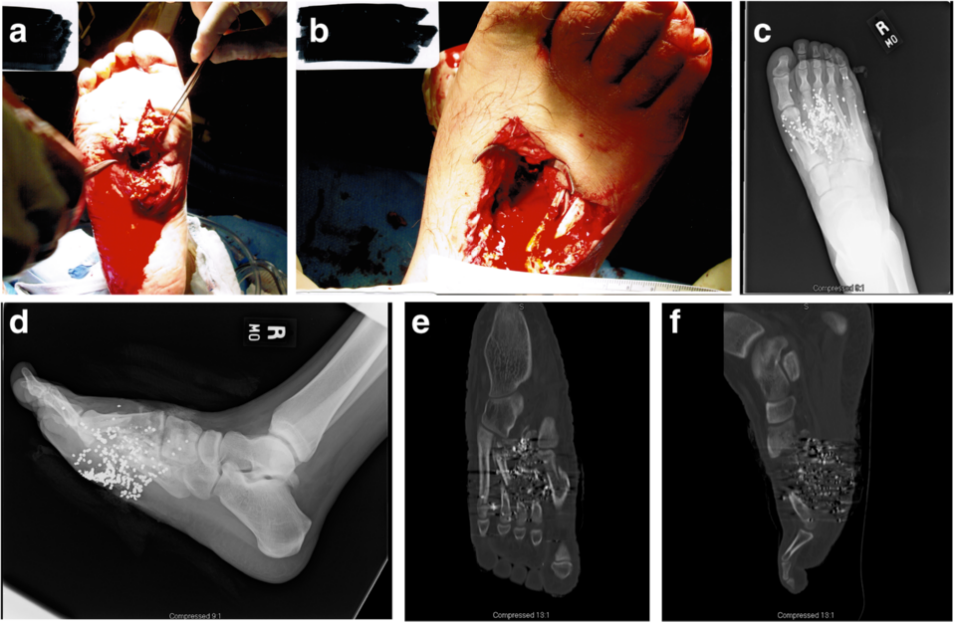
Photographs (a-b), radiographs (c-d), and CT (e-f) of the through-and-through shotgun injury to the right foot. Radiographs (c-d) and CT reconstructions (e-f) demonstrate significant buck-shot, first metatarsal fracture, comminuted 2^nd^ and 3^rd^ metatarsal fractures, fourth metatarsal fracture, fifth metatarsal neck fracture, and comminuted fractures of the intermediate and lateral cuneiforms (not shown).
